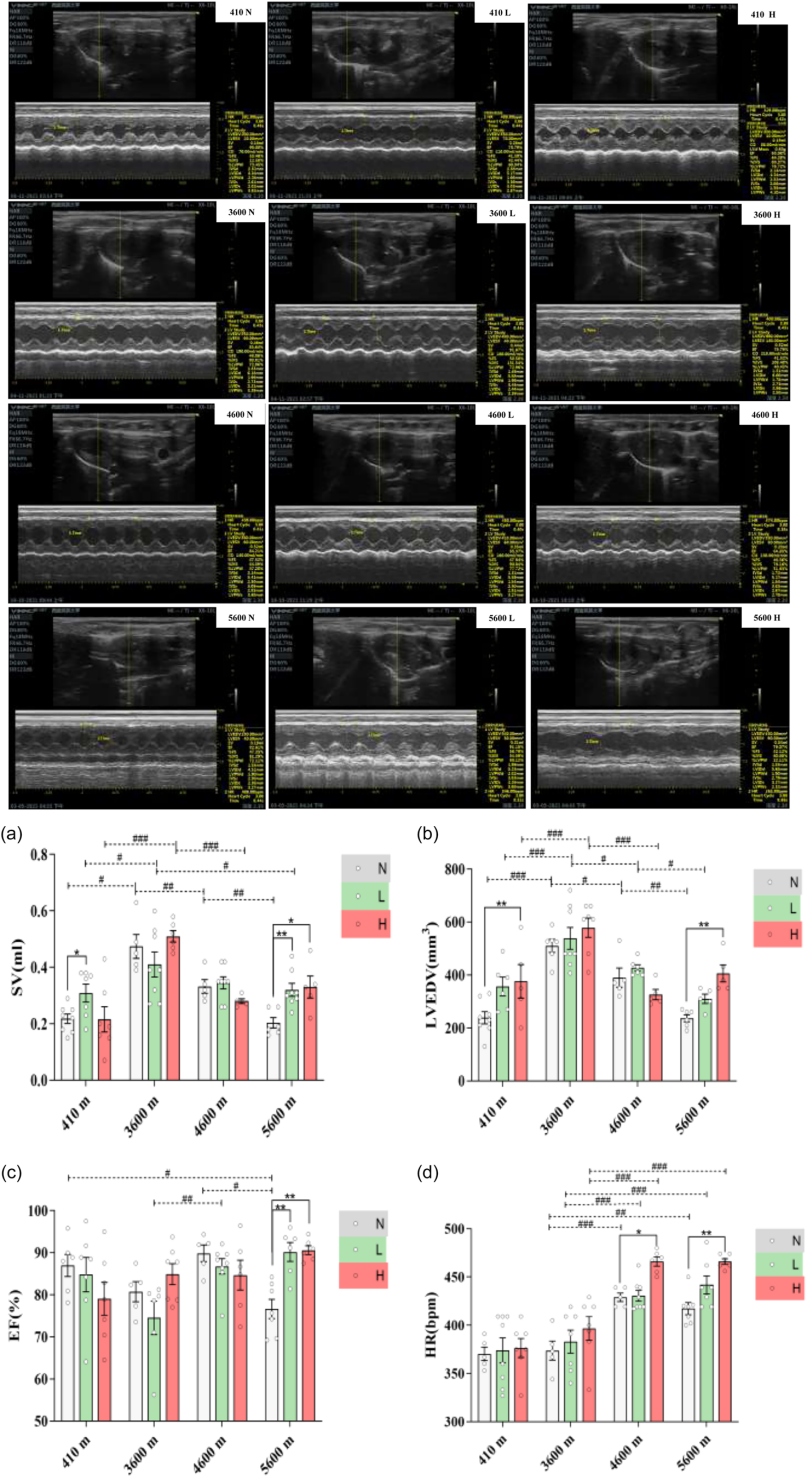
Echocardiograms and statistical analysis. (a) Stroke volume. (b) Left ventricular end‐diastolic volume. (c) Ejection fraction. (d) Heart rate. Data were analysed by one‐way ANOVA followed by the LSD test (c) or the Games–Howell multiple comparisons test (a, b, d) and are expressed as means ± SEM. P* < 0.05 and *P* < 0.01 comparing exercise intensities in same altitude; #*P* < 0.05, ##*P* < 0.01 and ###*P* < 0.001 comparing altitude conditions in same exercise intensities.
